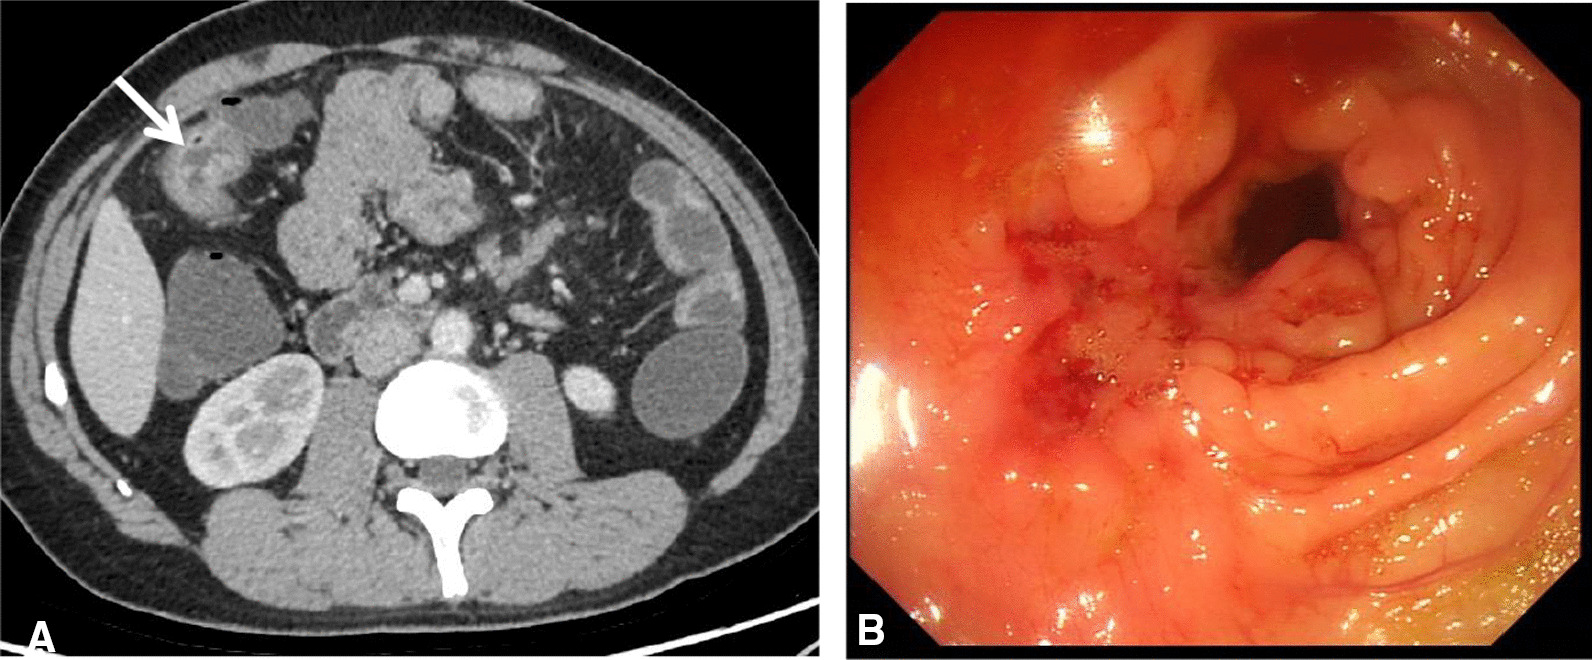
A 30-year-old woman with severe Crohn’s disease, A axial multidetector CT image of the transverse colon shows abnormal wall thickening, transmural enhancement (full arrow) and ulcer (white arrow) with CTEIA score 18; B view at ileocolonoscopy shows longitudinal ulcer in the same segment with CDEIS score 29
